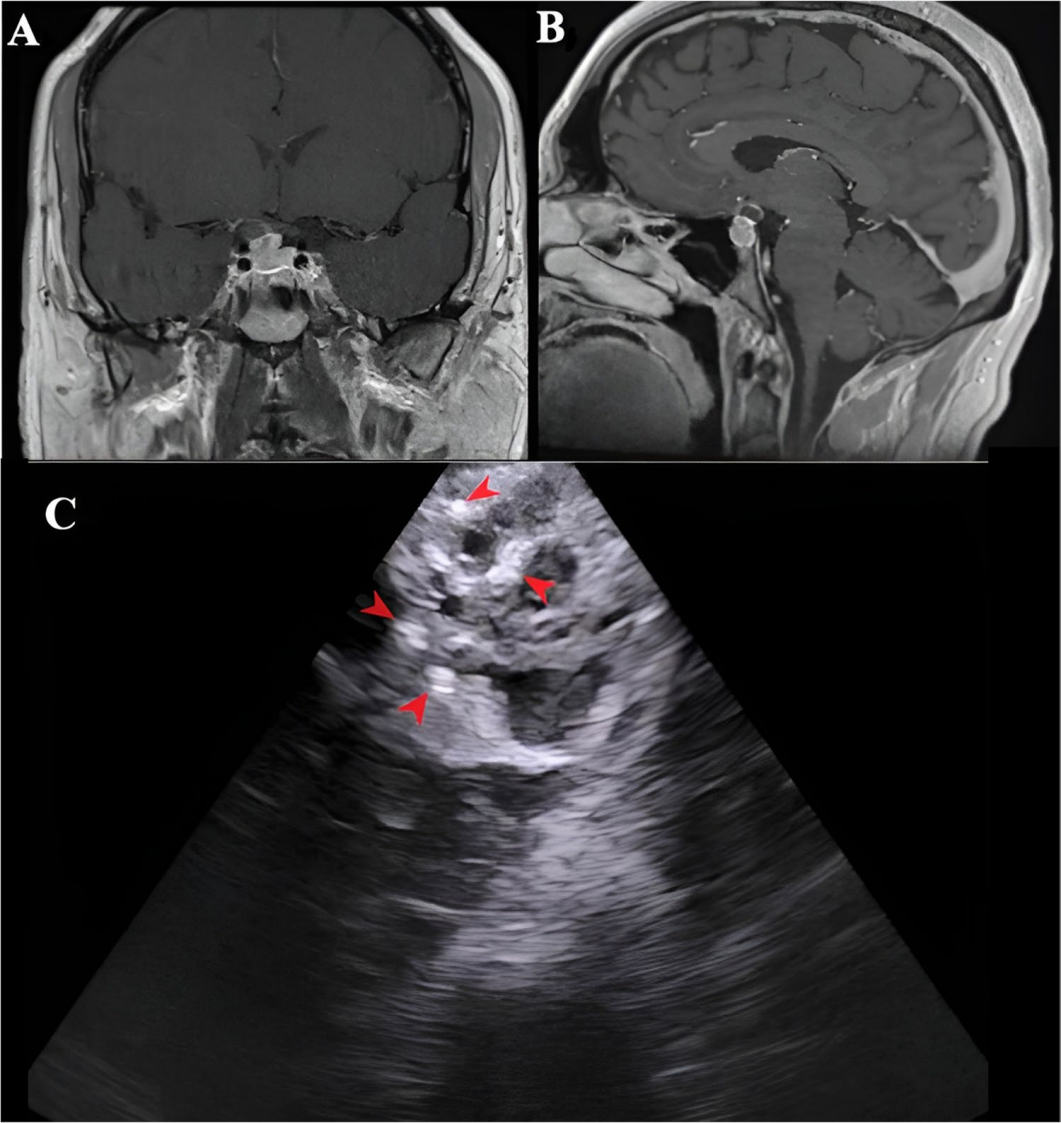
(A) and (B) Preoperative axial and coronal T1 with contrast MRI images of another case of ACP (fifth patient in the series), (C) Corresponding IOUS image showing the typical features with calcific foci (red arrowheads) This figure is original to this submission, so no credit or license is needed
